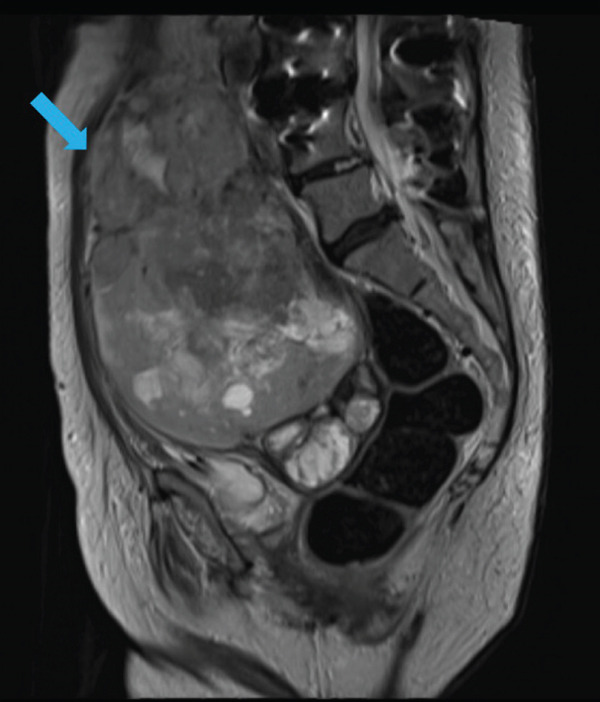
Sagittal T2‐weighted MRI demonstrating a large, heterogeneous uterine mass measuring 9.9 × 15.1 × 18.8 cm (blue arrow) with areas of necrosis and hemorrhage, consistent with primary uterine leiomyosarcoma.
